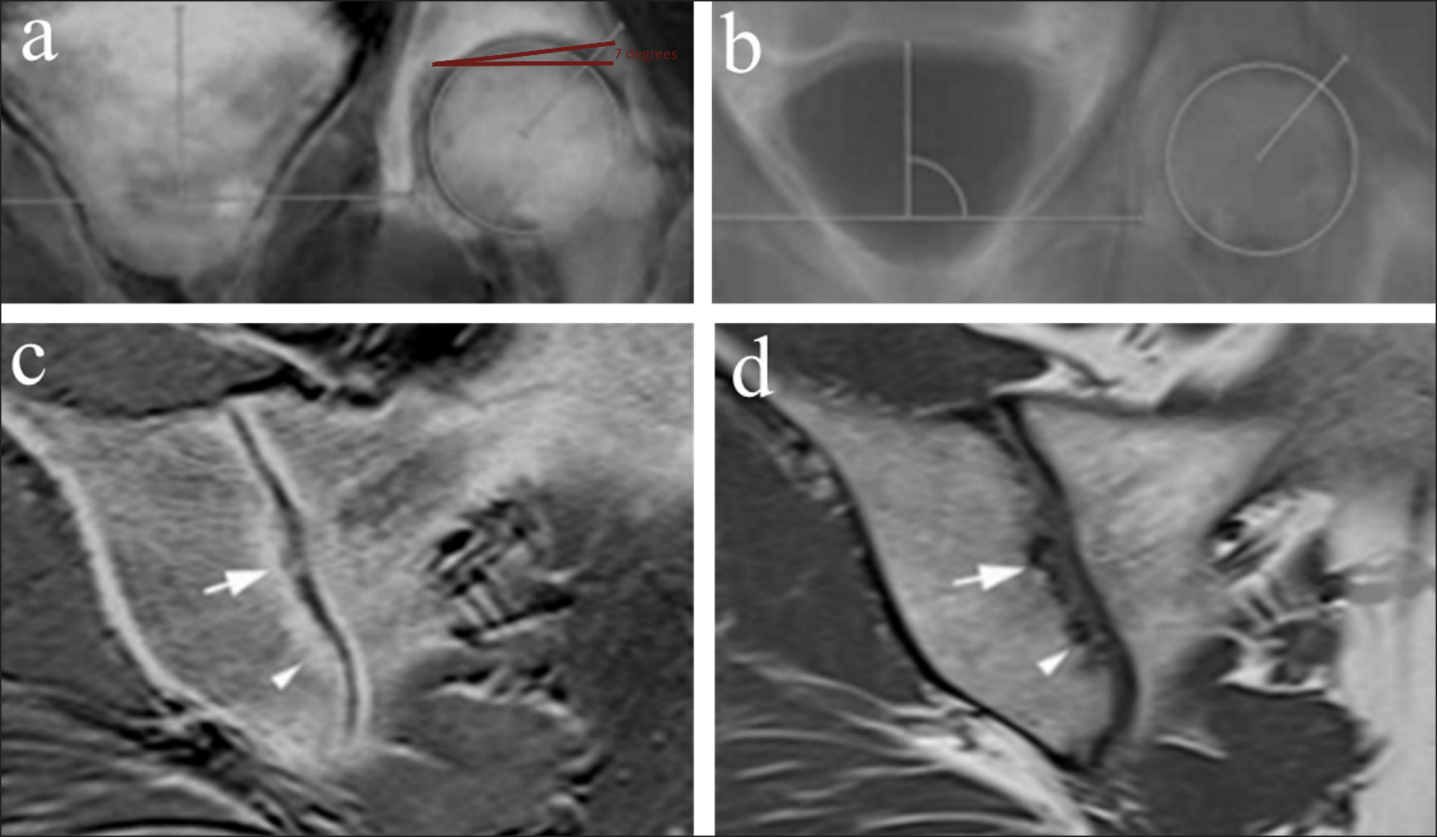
Susceptibility weighted images (SWI) versus T1-weighted (T1W) images. (a, b) Coronal maximum intensity projections of SWI MRI magnitude images (a) and T1W MRI (b) with magnifications and angle measurements. The acetabular index or Tönnis angle (red lines), measurement of acetabular inclination, is an angle assessed by a line drawn from the medial edge of the acetabular sourcil through to the most lateral aspect of the sourcil and by a second line drawn in the horizontal plane of the pelvis (normal range 3°–13°, in this case 7°). This angle could not be measured on T1 because the acetabular sourcil could not be identified reliably. Figure reproduced from Böker et al. [21] under the Creative Commons Attribution 4.0 International License (CC Creative Commons — Attribution 4.0 International — CC BY-NC 4.0, https://creativecommons.org/licenses/by-nc/4.0/). Minor modifications were performed for presentation purposes. (c, d) False positive detection of erosion (arrow) in T1 in the sacroiliac joint of a 51-year-old woman with spondylarthrosis. SWI (c) shows smooth joint surfaces with mild sclerosis mimicking erosive changes (arrowhead) in T1 (d). Figure reproduced from Deppe et al. [20] under the Creative Commons Attribution 4.0 International License (CC Creative Commons — Attribution 4.0 International — CC BY-NC 4.0, https://creativecommons.org/licenses/by-nc/4.0/). Minor modifications were performed for presentation purposes.
